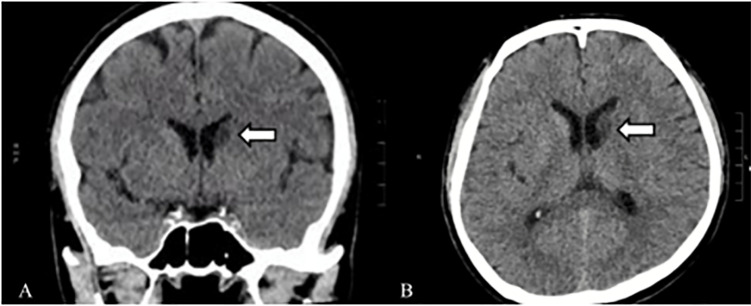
Coronal images of a brain computed tomography without contrast of a 58-year-old male presenting with a headache, hand numbness, and phantosmia showing a hypodensity in the left caudate nucleus in the (A) coronal (arrow) and (B) axial (arrow) views.
